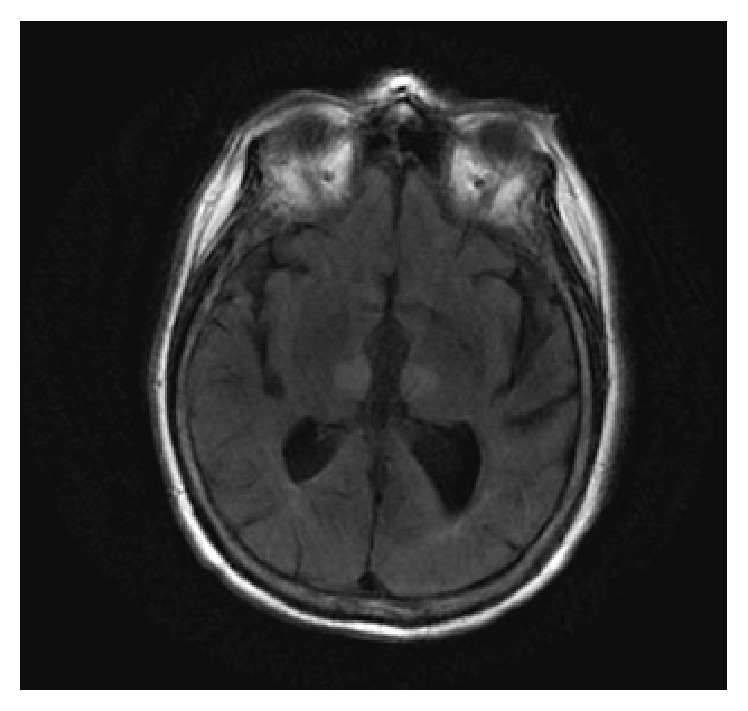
MRI with fast fluid-attenuated inversion showing hyperintensity at bilateral thalamic areas.
